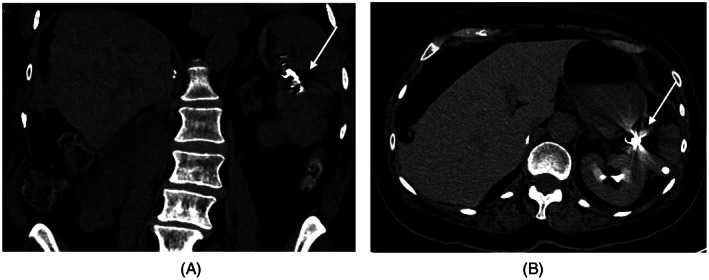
CT SCAN images were obtained post coiling (arrow) of the ruptured splenic artery aneurysm (A) Coronal image; (B) Axial image
